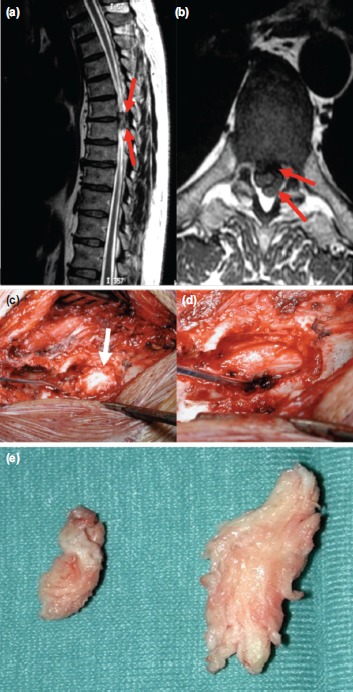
(a,b) T2-weighted MRI images of the thoracic spine that showed a large herniated disc fragment migrated posteriorly that caused cord compression. The severity of the cord compression was more evident on the axial plane. Intraoperative images after posterior laminectomy (c) before and (d) after herniated disc removal. (e) Macroscopic image of the fragment of disc surgically removed.
